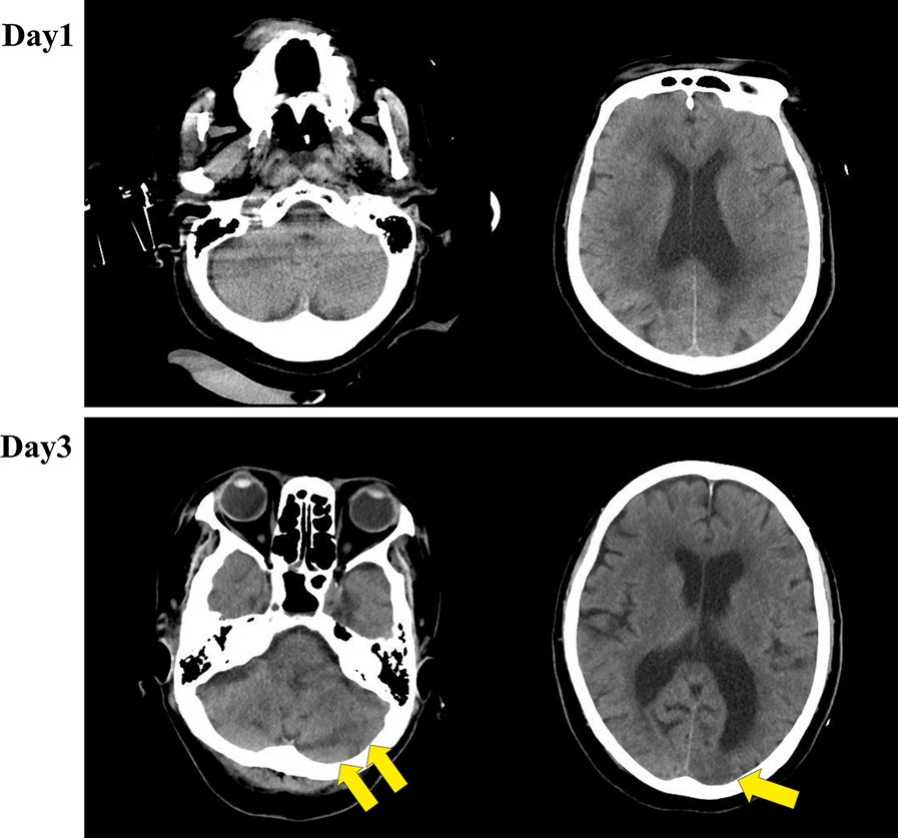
Head computed tomography on days 1 and 3. Head computed tomography (CT) examination on day 1 (upper panels) and day 3 (lower panels). No abnormal lesion is found on day 1. On day 3, low-intensity areas are detected in the cerebellum and left occipital lobe (yellow arrows).
